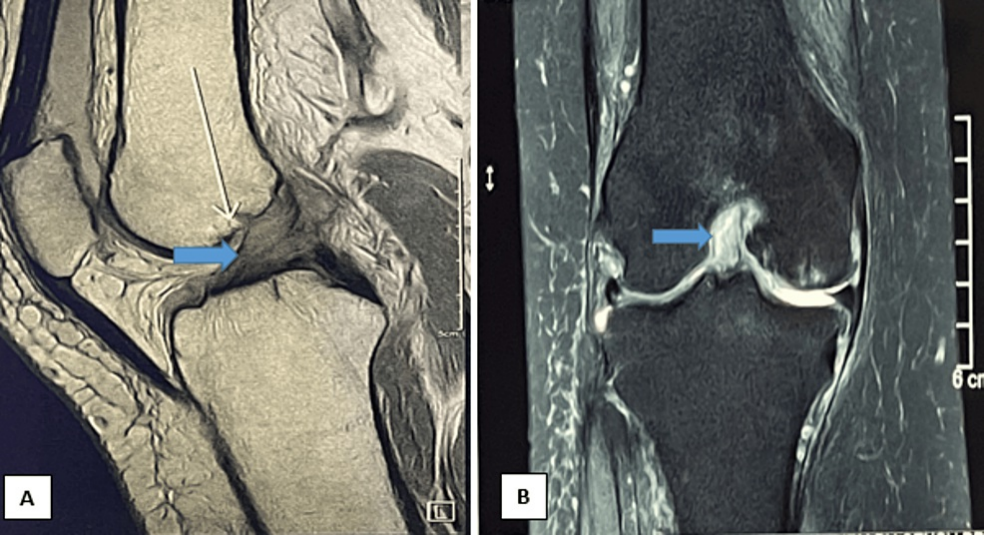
MRI of the knee at presentation A: MRI Sagittal T1-weighted image showing the high signal intensity of the anterior cruciate ligament with a "celery stalk" appearance. B: MRI Coronal T2-weighted image showing a thickening of the anterior cruciate ligament with meniscus lesions MRI: magnetic resonance imaging
